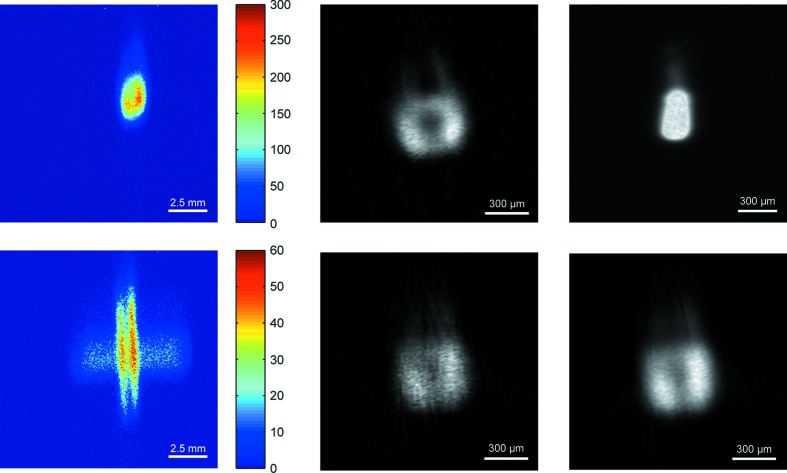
Images of a focused monochromatic (12 keV) beam as measured at bending-magnet beamline B16 (DLS, UK). Top row: a raw unprocessed XBI image obtained using a 200 µm circular aperture, *L*/*D* = 3 and counting time of 0.5 s (left); corresponding reconstructed beam cross-section image (centre); reference direct image taken with an X-ray Eye camera set up 20 cm downstream and near the focal point (right). Bottom row: a raw XBI image obtained using a cross aperture with slit size of 1700 µm × 25 µm, *L*/*D* = 3 and counting time of 1 s (left); corresponding reconstructed beam cross-sectional image (centre); beam cross-sectional image reconstructed from an image obtained by averaging ten raw XBI images (right). Beam cross-sectional images were reconstructed using 16 Lucy–Richardson iterations. Other XBI parameters: 125 µm Kapton foil, α = 25°, noiseless Medipix-2 detector with 55 µm pixels (Llopart *et al.*, 2002 ▶).
